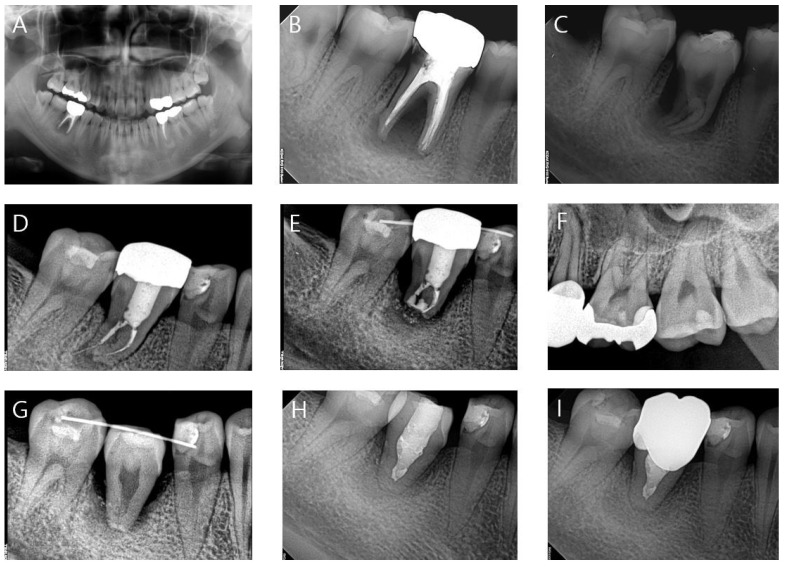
(A,B) Panoramic and periapical view at first visit. Periapical view immediately (C) and 7 years (D) after first autogenous tooth transplantation from #48 to #46. (E) 2 months after root resection (F). Periapical view of donor site (#28). Periapical view after 1 week (G), 3 months (H) and 18 months (I) after second autogenous tooth transplantation from #28 to #46.
